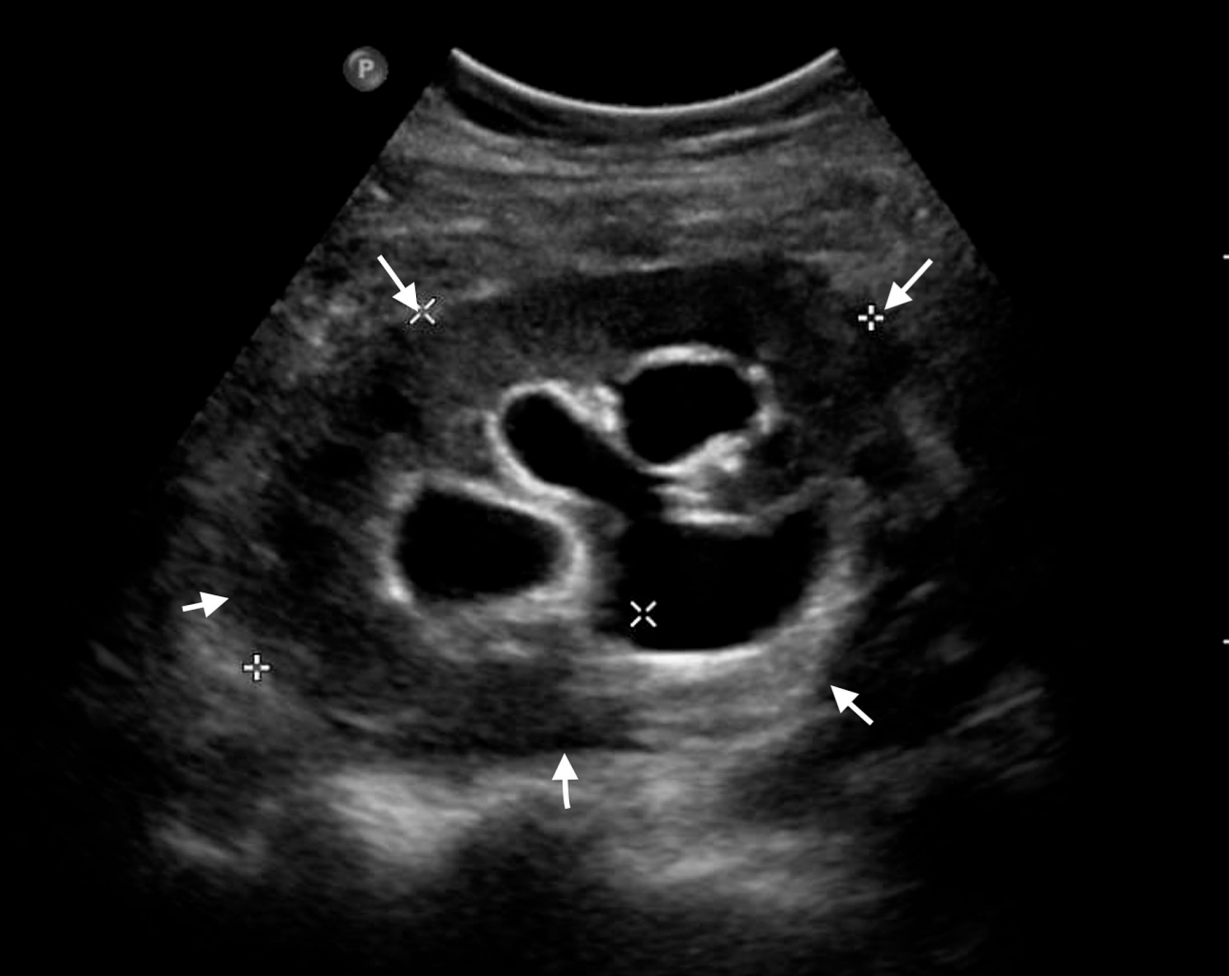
Ultrasound of transplanted kidney Transplanted kidney is seen in the right iliac fossa measuring 9.4 cm x 5.9 cm x 5.4 cm with volume of 160 ml (white arrows). It shows normal echogenicity and cortical thickness with mild hydronephrosis and trace of perinephric fluid. No evidence of anastomotic stenosis. Duplex parameters are all within normal limits.
